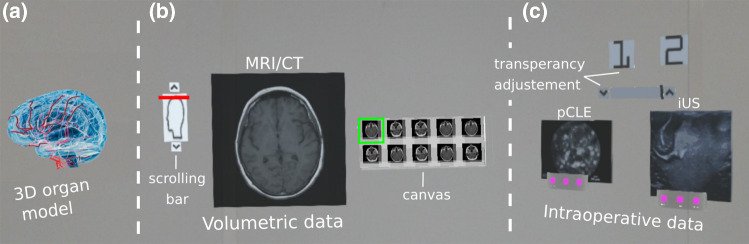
Prototype of the scene with the three MR components. These components include a 3D organ model (a), a volumetric imaging data component (b), and a component to visualise intraoperative data (c). Here, the volumetric data show the MRI data of a brain glioblastoma [14], and the 3D model was adopted from [15]
